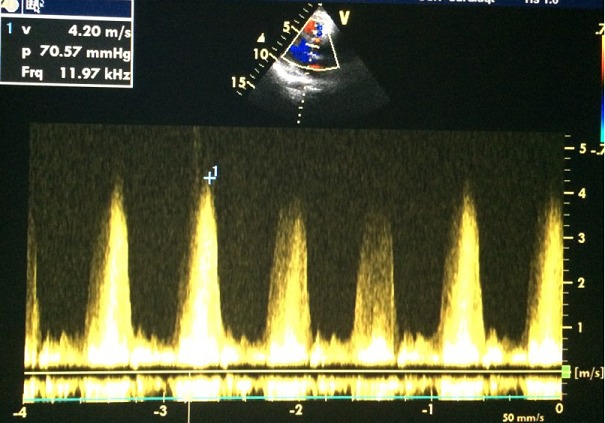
Flux restrictif de la CIV au Doppler continu
